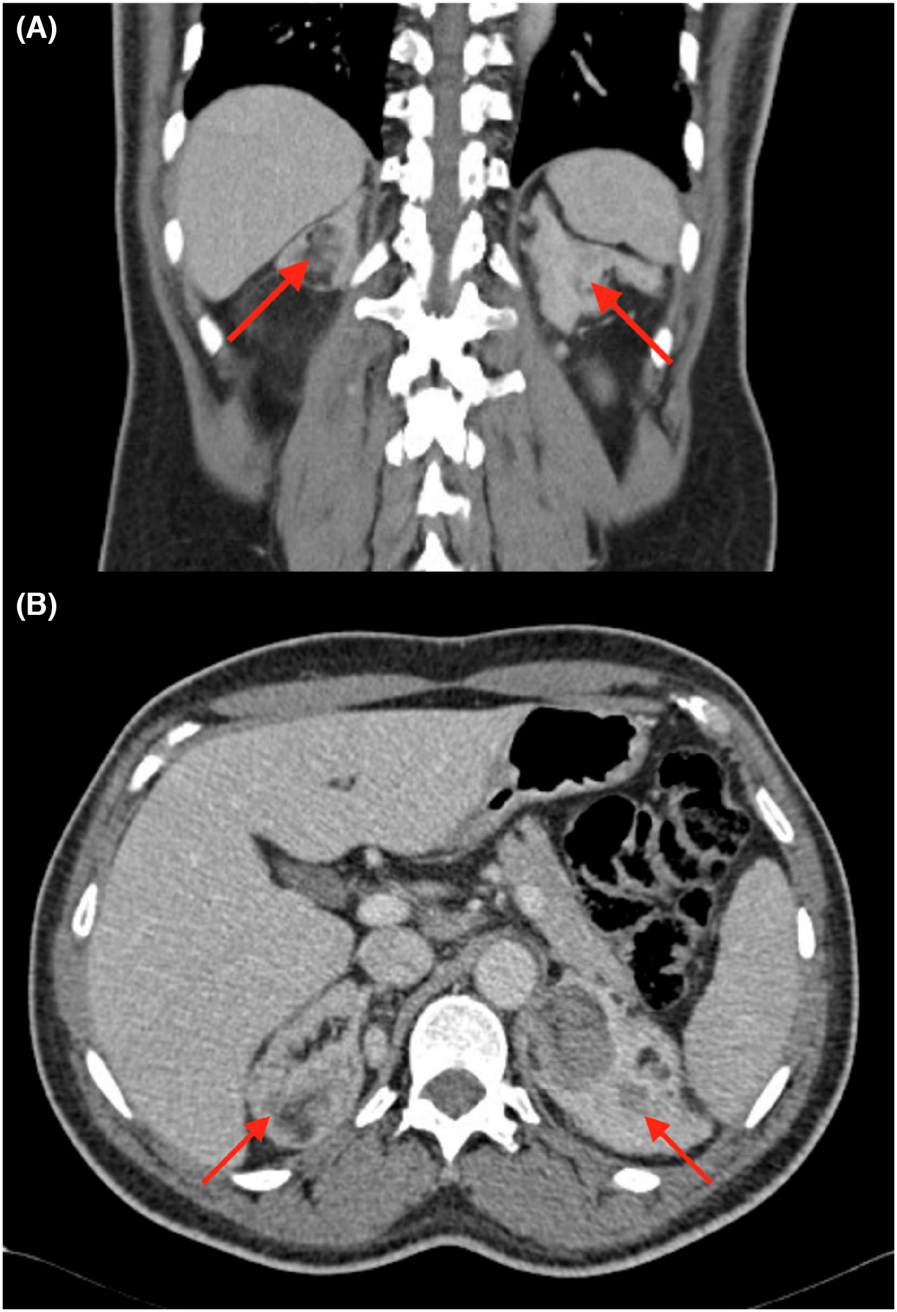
On the abdominal computed tomography (A: coronal view, B: axial view), the red arrows show a bilateral enlarged adrenal glands associated with voluminous lesions with lipomatous density
